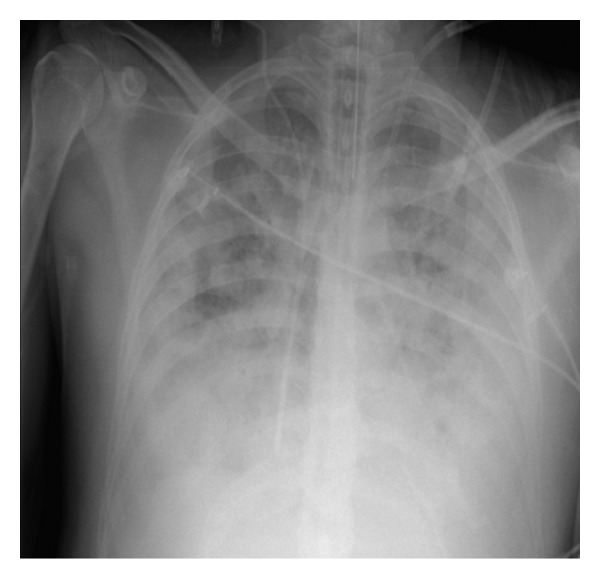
Last chest X-ray at the ICU, showing worsening diffuse bilateral nodular and patchy infiltrates.
